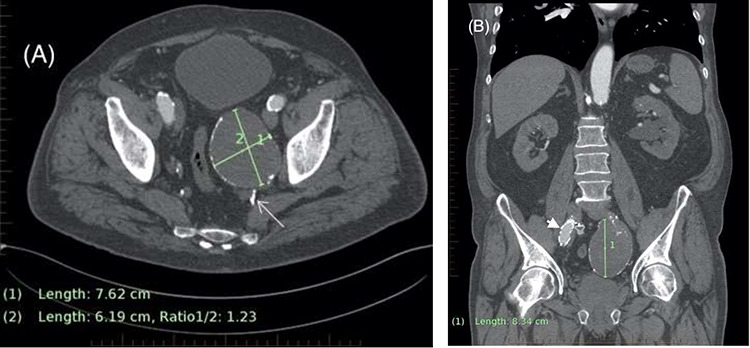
(A) Axial CT demonstrating left internal iliac aneurysm (IIAA) measuring ×76 62 mm with retrograde flow into the aneurysm (arrow) from the deep pelvic vessels. (B) Coronal CT demonstrating the left IIA with a craniocaudal length of 83 mm. The right external iliac artery endograft limb is also visible (arrowhead).
